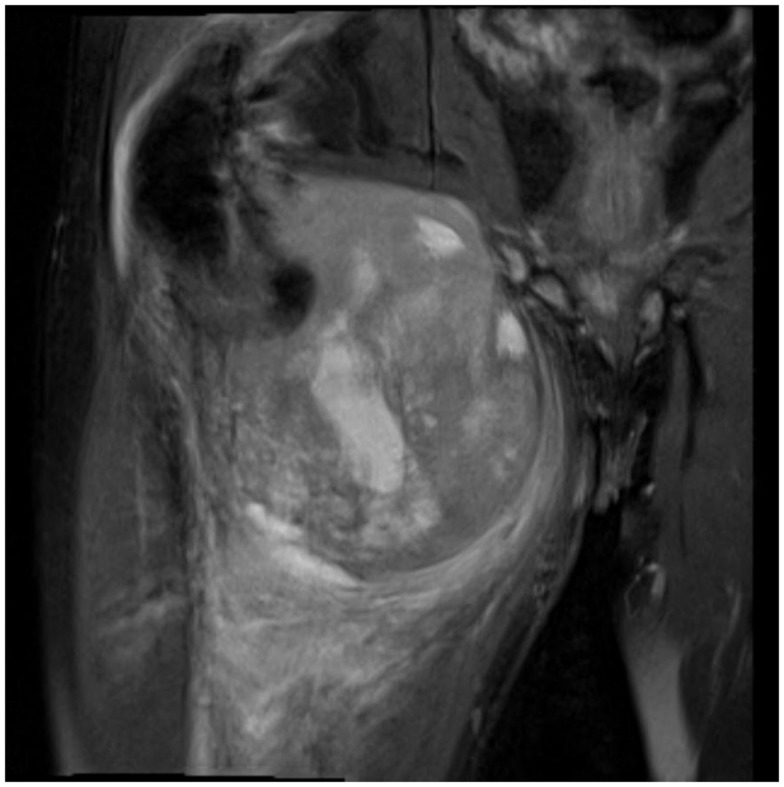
MRI of a soft-tissue sarcoma of the thigh, reaching up to the pelvic bones.
